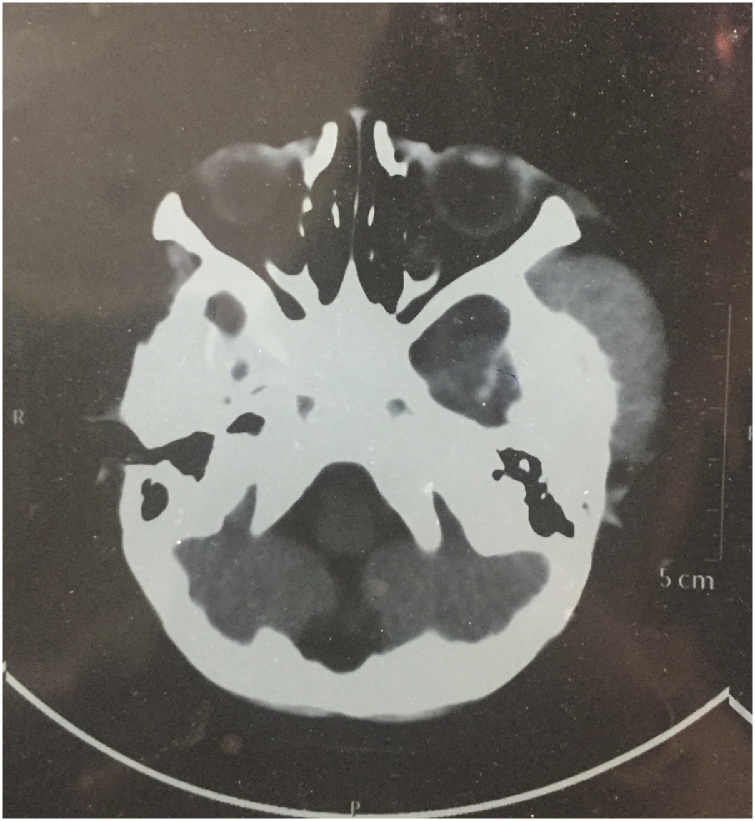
On MRI, a lesion measuring 5 × 3 cm in left temporal region eroding the underlying bone.
